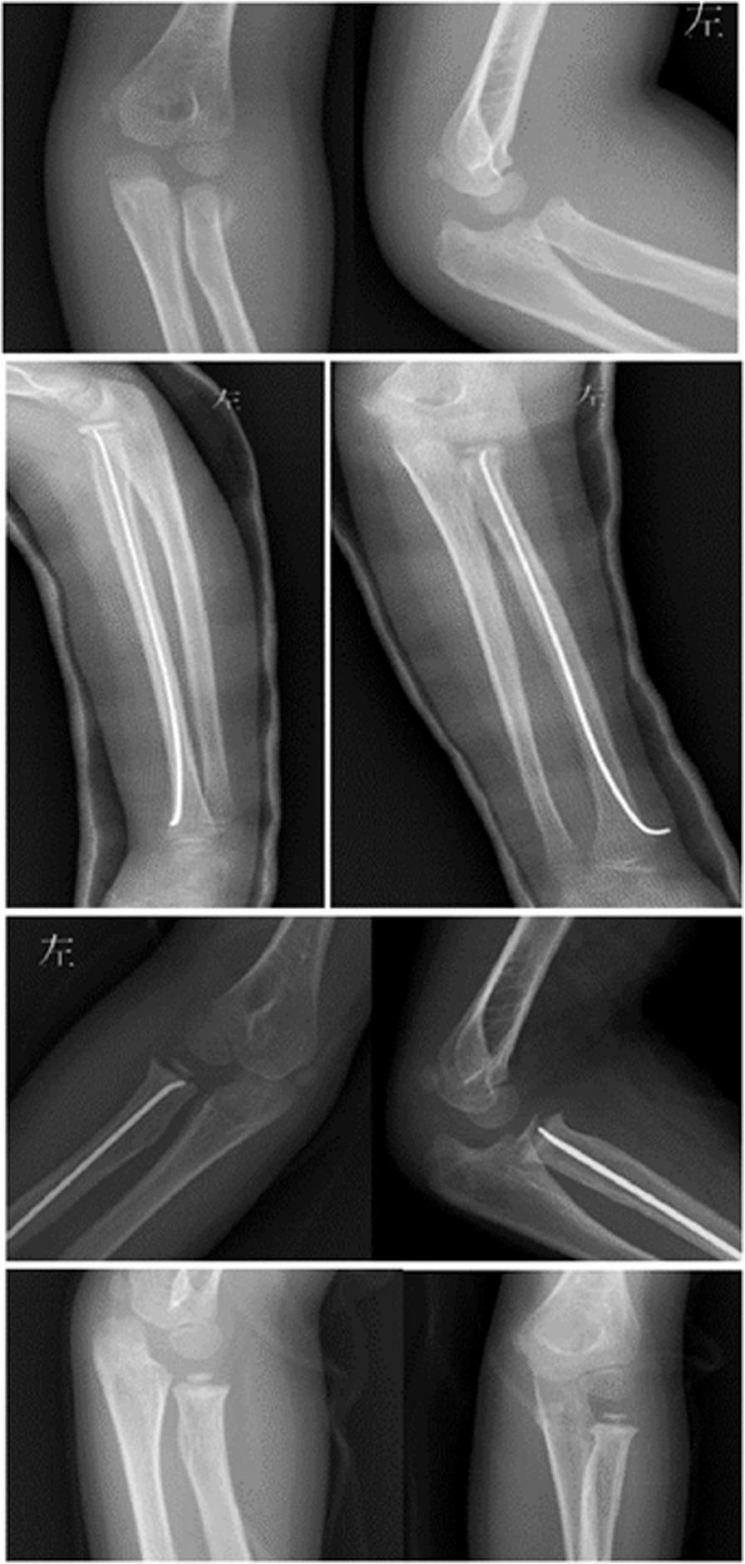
preoperative x-ray, postoperative and final follow-up X-rays of patient No. 6
